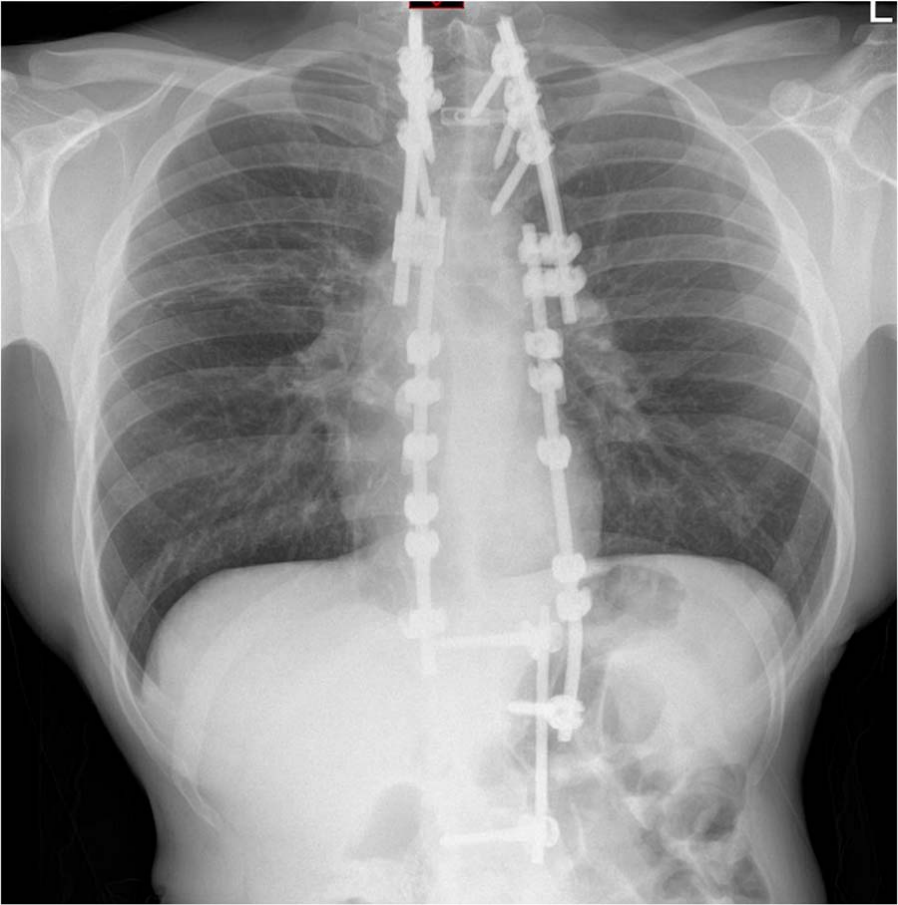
Repeat chest radiograph on re-admission to hospital with fevers and tachycardia.
